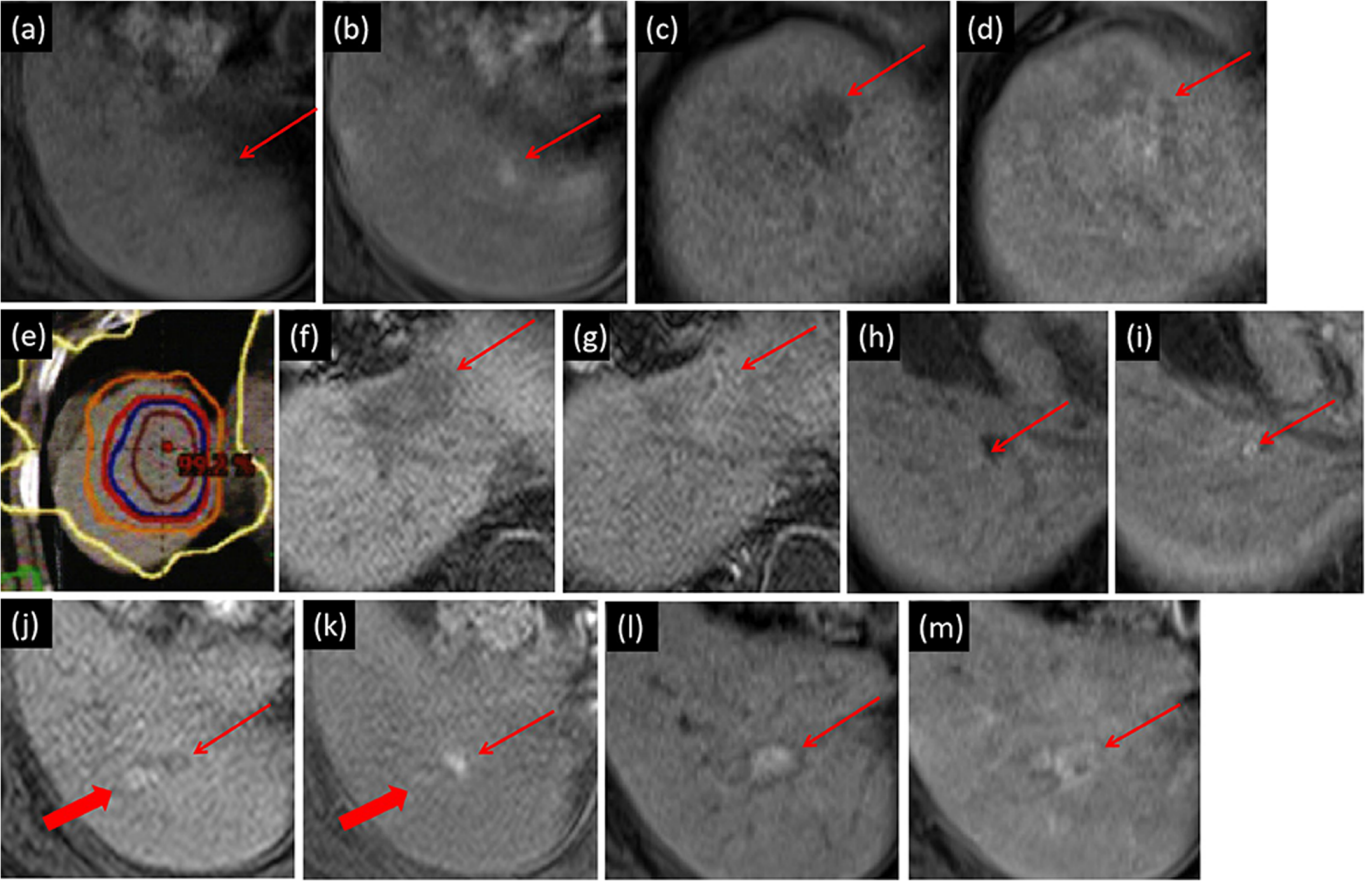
A case of progressive tumor disease and local tumor progression. (a,b) RFA was planned for an 11 mm lesion in segment (S)6, showing unenhanced T1-weighted image and AP of EOB-MRI, respectively. The lesion was hyperenhanced. (c,d) A 32 mm lesion located in S8 was visible in both the T1-weighted image (c) and the AP of the EOB-MR image (d). Because of blind areas hidden in the lung gas, RFA treatment was impossible. (e) A dose distribution picture of SBRT treatment plan was generated. As this lesion was located adjacent to the gastrointestinal tract, radiotherapy with mild hypofractionation was performed. The total dose of 42Gy (red isodose line) was delivered in 14 fractions. The central part received 60 Gy radiation. (f,g) Compared with the unenhanced T1-weighted MR image (f), at the one-year follow-up, the SBRT-treated area changed to isoenhancement (g). There was a slight liver deformation caused by radiation irritation due to SBRT treatment. (h,i) About one month after RFA treatment, in the unenhanced T1-weighted image (h) and the AP of the EOB-MR image (i), the ablation area showed scars from treatment but no appearance of recurrence. (h–k) However, a T1-weighted image was taken (h) as a reference at the 4 months’ follow-up. A hyperenhanced area, just adjacent to the initial ablated area (thick arrow, hyperenhanced scars of ablated trace), was found in the AP of the EOB-MRI (k). The local recurrence was repeatedly treated by RFA. (l,m) Two months after the second RFA treatment, the ablated area appeared as hyperenhanced scars in both the unenhanced T1-weighted image (l) and the AP of the EOB-MRI (m). Thin arrows in (a–d) and (f–m) indicate the location of the target lesion or post-treated area. This case corresponds to the No. 12 patient shown in the three tables
